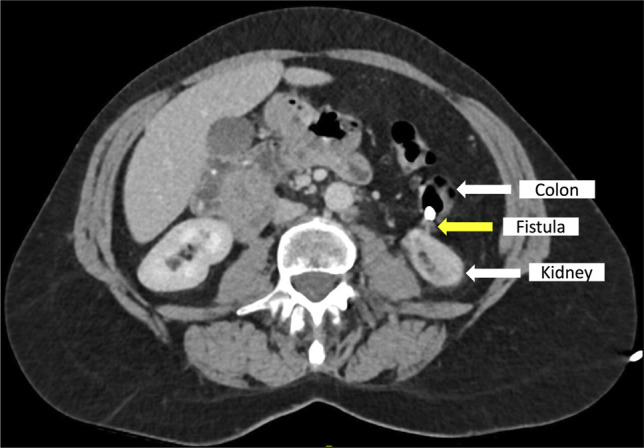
Radiographical closure of the left-sided colorenal fistula demonstrated on computed tomography scan in October 2025, with no air visualized in the fistula tract.
